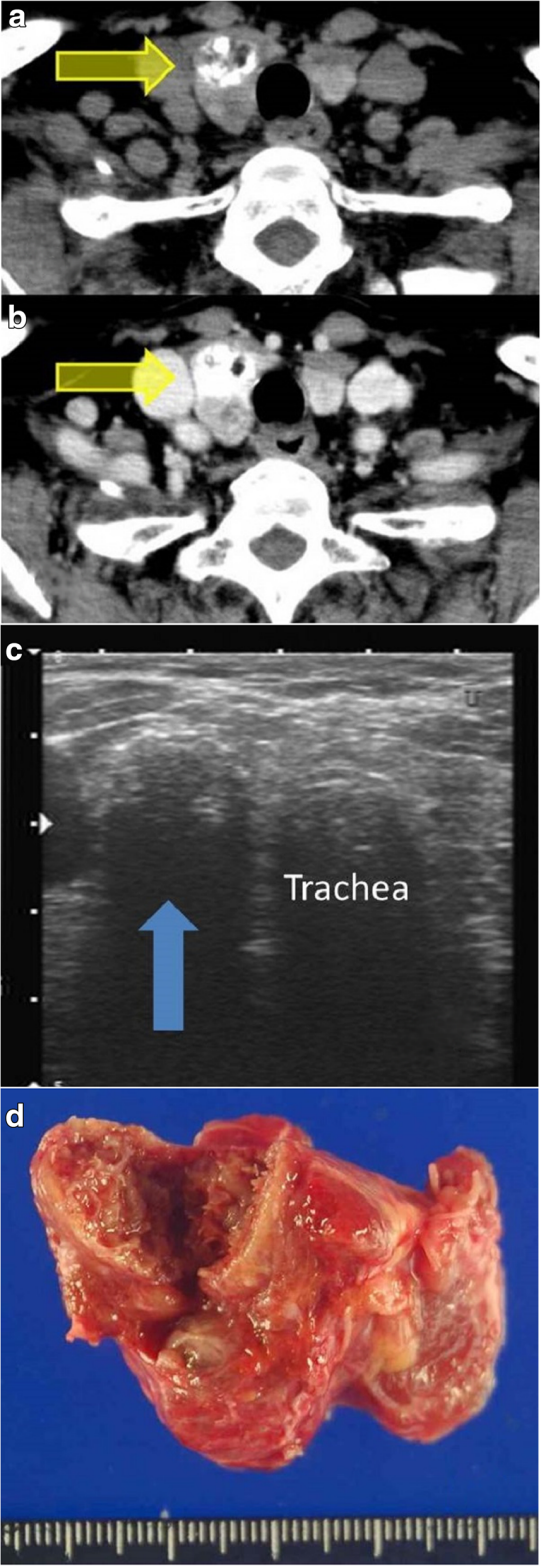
Characteristics of the thyroid nodule. Computed tomography scan showing the right thyroid nodule with calcification (*arrow*) (a). The nodule is enhanced and shows no signs of extrathyroidal invasion (*arrow*) (b). Ultrasonogram showing the right thyroid nodule (21 mm in diameter) with calcification (*arrow*) (c). Macroscopically, a well-circumscribed mass in the right lobe of the thyroid is observed (d)
